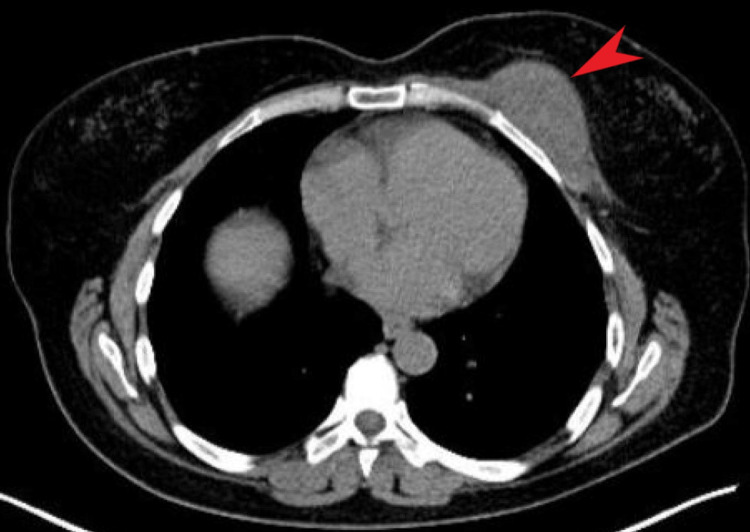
Positron Emission Tomography Chest Imaging In this section of PET-CT, a mass in the left chest can be seen (red arrow), with suspicion for minimal invasion of the underlying bone structures and intrusion into the breast tissue.
